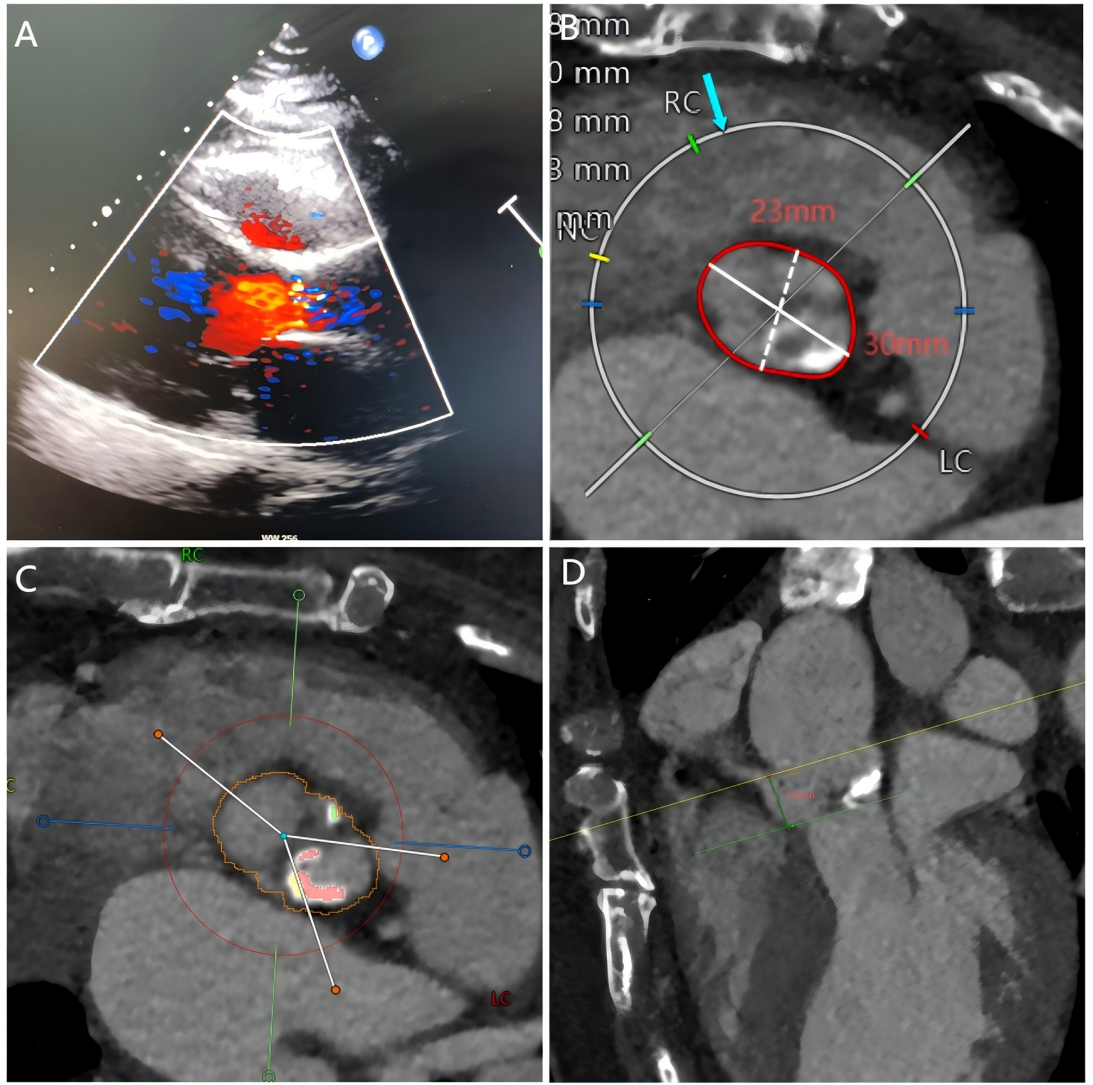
Echocardiography and cardiac computed tomography prior to TAVR. (A) Echocardiography showed severe aortic stenosis. (B–D) Pre-TAVR CT showed the aortic annulus diameter, calcified bicuspid aortic valve, and the position of the right coronary artery.
